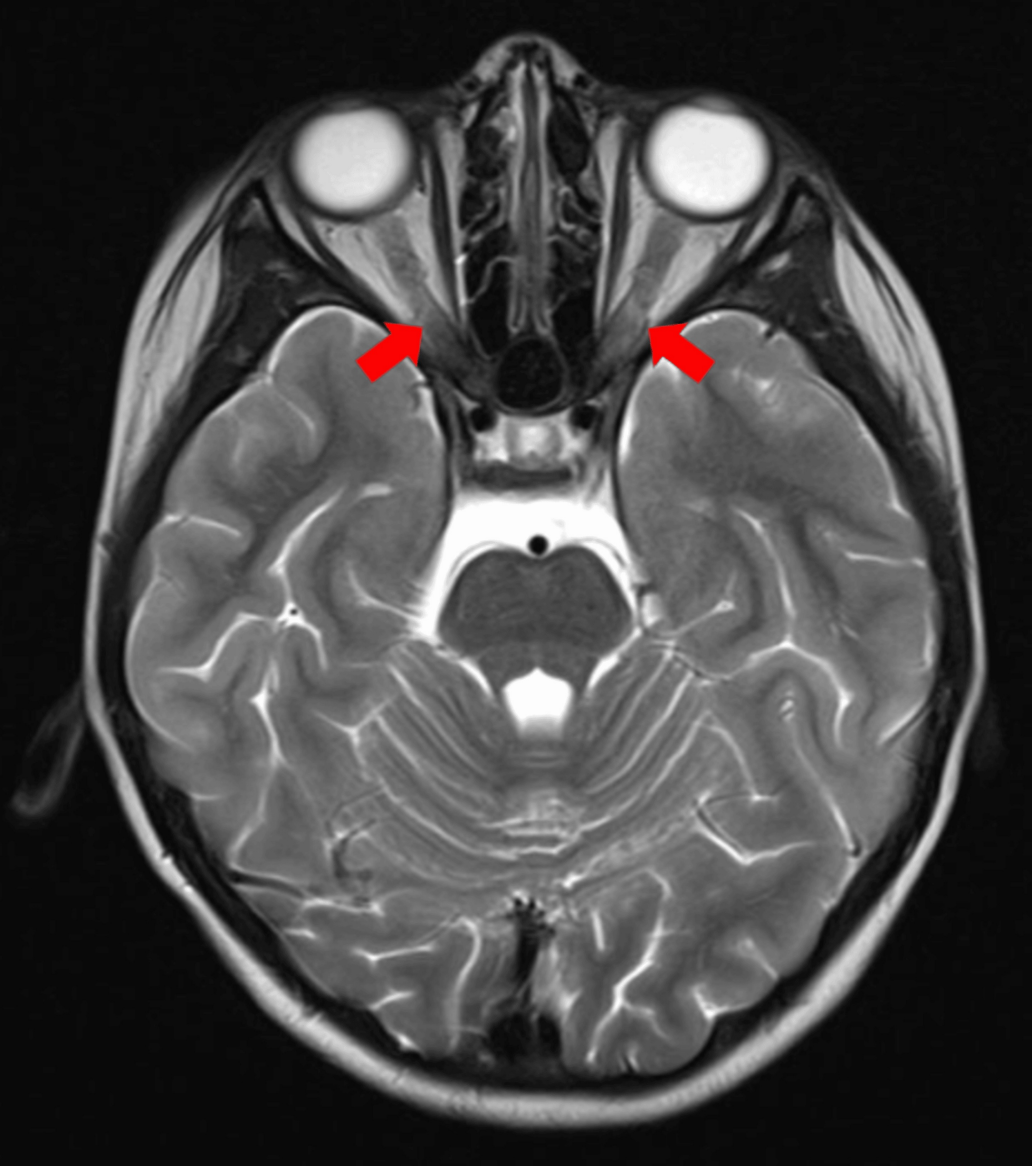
MRI brain and orbit T2-weighted post gadolinium showing symmetrically thickened optic nerve till the optic chiasm (red arrow)
